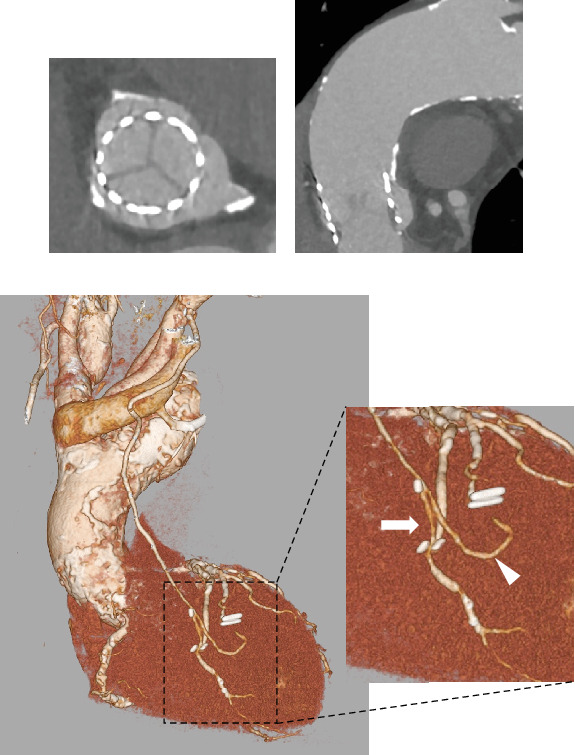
Follow-up coronary computed tomography angiography after transcatheter aortic valve implantation and minimally invasive cardiac surgery–coronary artery bypass grafting. (a) A short-axis CT image of the 26-mm Sapien 3 balloon–expandable THV, (b) a long-axis CT image of the THV, and (c) the LITA-LAD (arrow) and LITA-SVG-Dg (arrowhead) are both patents. Abbreviations: CT, computed tomography; Dg, diagonal branch; LAD, left anterior descending coronary artery; LITA, left internal thoracic artery; SVG, saphenous vein graft; THV, transcatheter heart valve.
